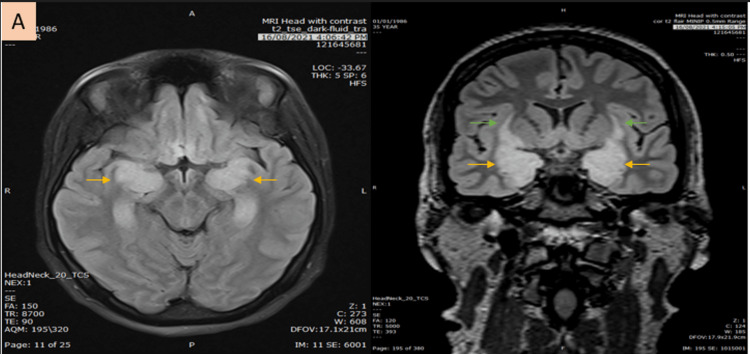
Axial and coronal head MRI T2/FLAIR show symmetrical swollen bilateral hippocampal (yellow arrow) and claustrum (green arrow) with T2/FLAIR bright signal. The same regions of both hippocampi and claustrum demonstrate T1 relatively low signal and no significant contrast enhancement (not shown). MRI: magnetic resonance imaging; FLAIR: fluid-attenuated inversion recovery
